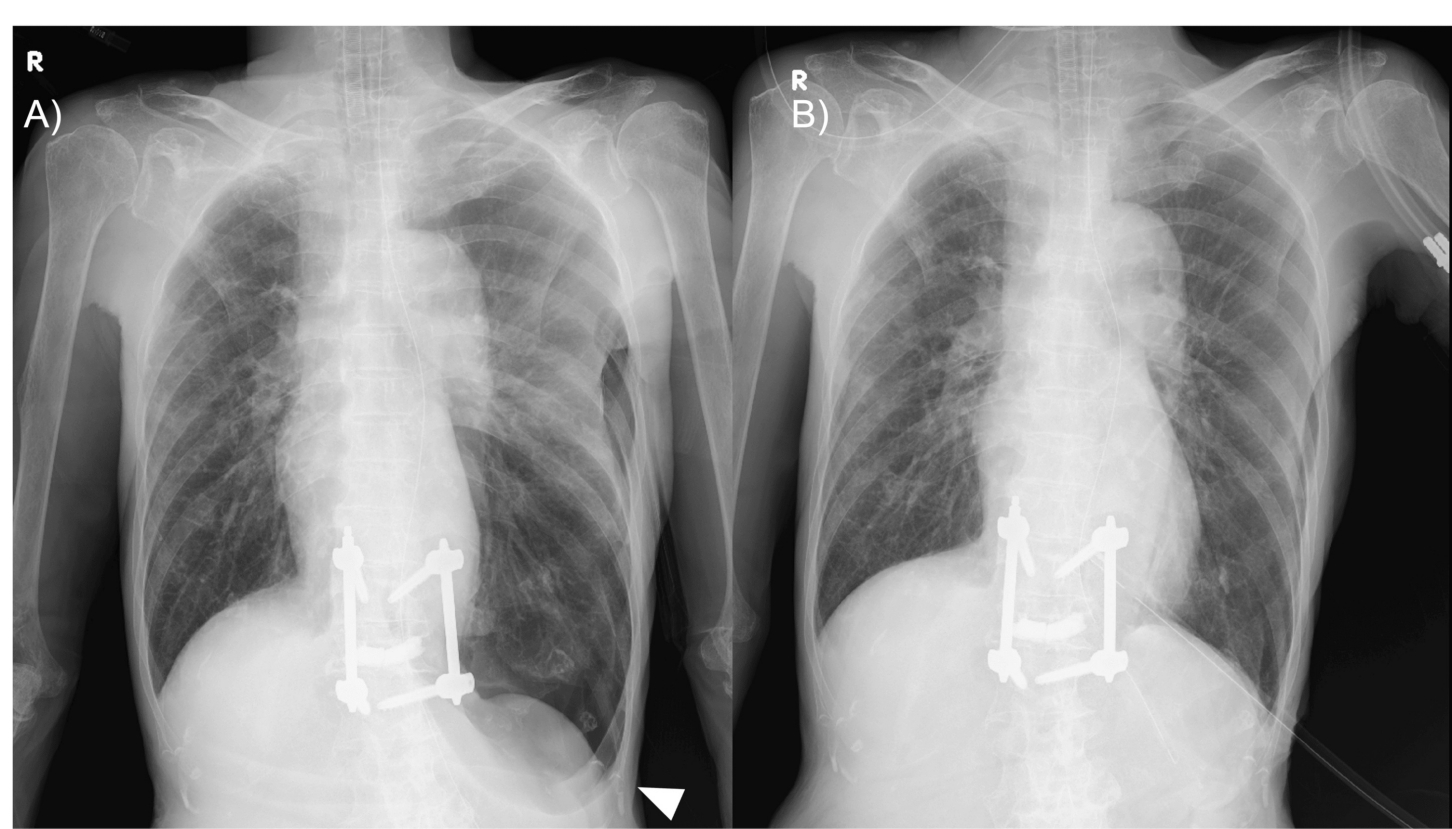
Postoperative and Follow-up Chest Radiographs Postoperative chest radiograph (A) showing a left-sided pneumothorax. Follow-up chest radiograph after chest tube treatment (B) confirms resolution without recurrence.
